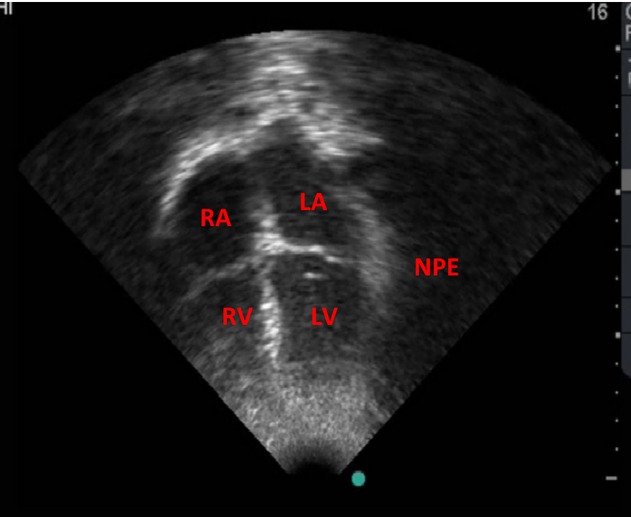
Apical Four Chamber view after pericardiocentesis showing expansion of the Chambers and absence of the pericardial effusion. (NPE, No pericadial effusion).
